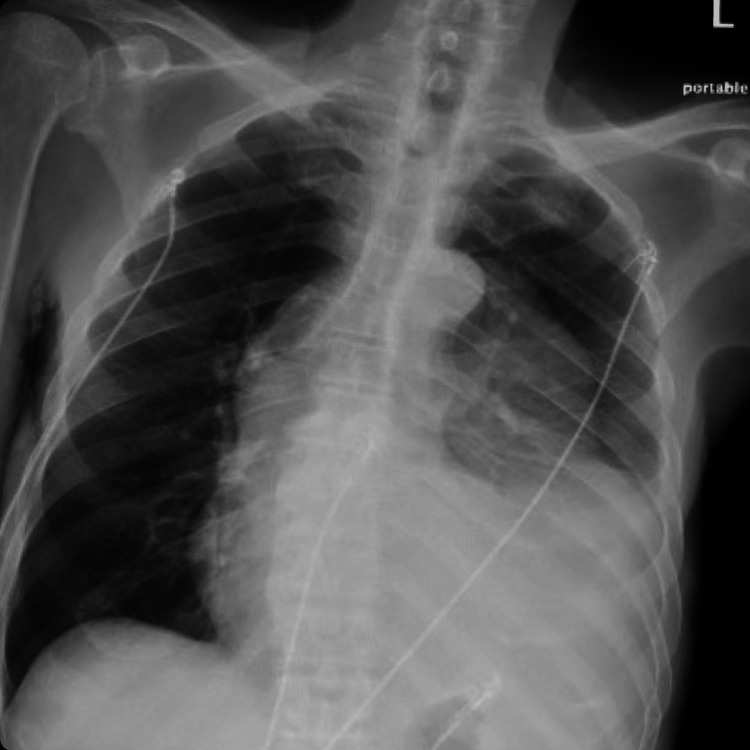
Chest X-ray showing pneumonia as well as abnormal mass in the left suprahilar region
